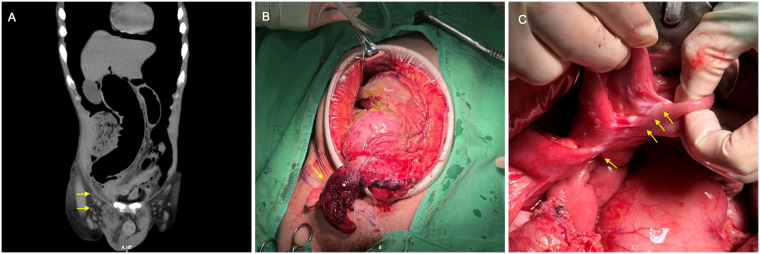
(A) A coronal computed tomography scan shows a loop of the transverse colon herniated into the right inguinal canal (yellow arrow), with associated marked dilatation of the ascending colon and cecum; (B) An intraoperative view demonstrates the incarcerated transverse colon within the right inguinal hernia sac, with ischemic necrosis and severe cecal dilatation (yellow arrow); (C) An intraoperative view reveals multiple fibrotic plaques on the mesentery (yellow arrow), with associated mesenteric shortening and tethering of small-bowel loops consistent with sclerosing mesenteritis.
